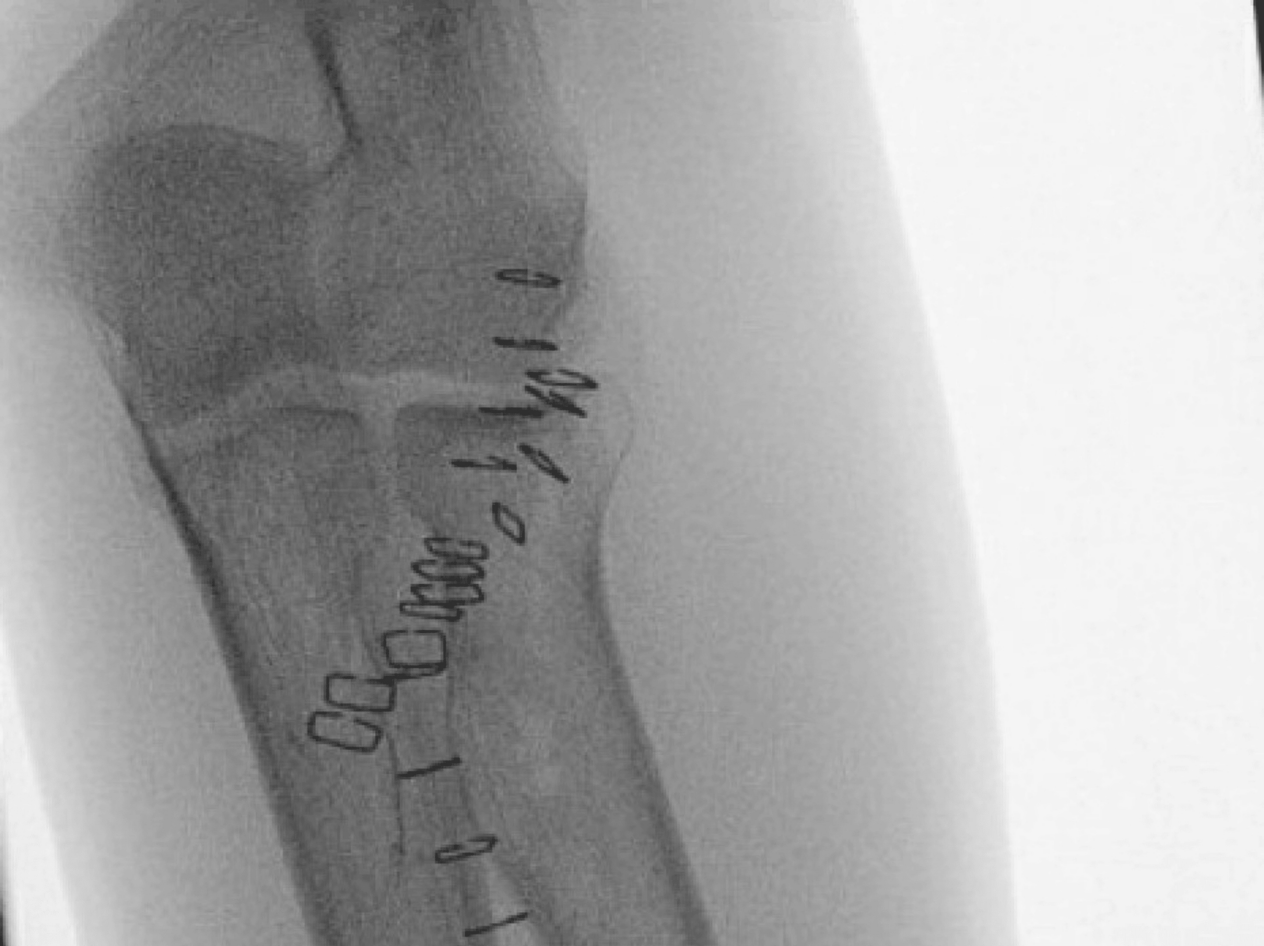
X-ray image of the patient’s left forearm. X-ray image of the patient’s left forearm, showing through-and-through tract in the radius with no obvious fracture.
